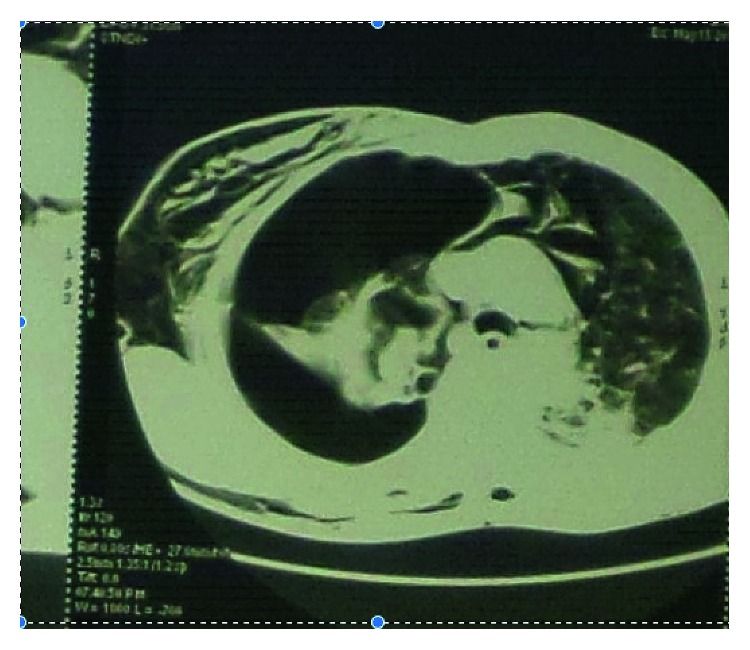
Chest CT scan transverse view. The aspergilloma cavity is seen at the apex of the right lung. There are massive pneumothorax with subcutaneous emphysema and bilateral lung fibrosis as sequelae of tuberculosis.
